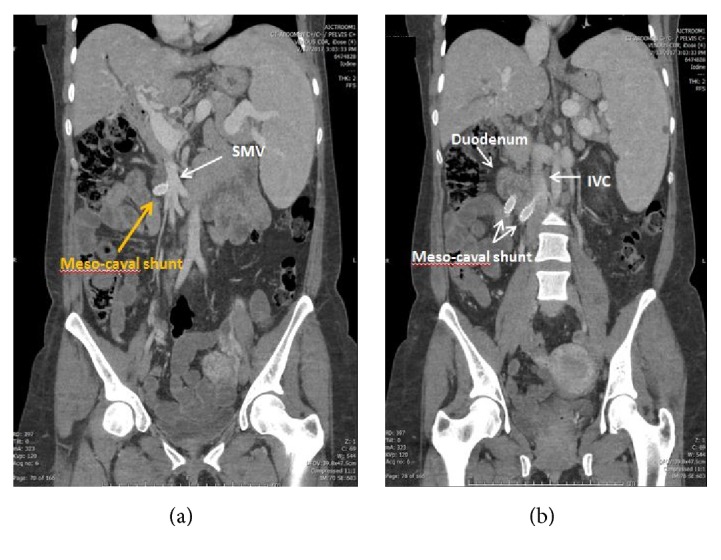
Coronal view on computed tomography showing the mesocaval shunt (yellow arrow) originating from the SMV and inserting into the infrarenal IVC.
